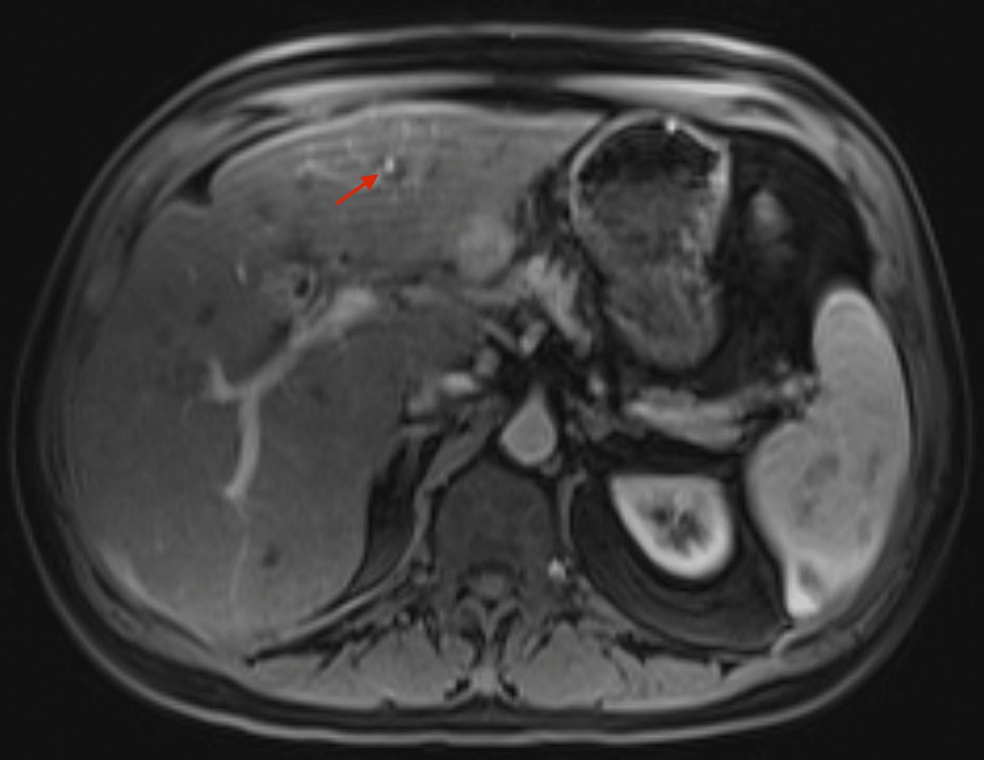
MRI image Magnetic resonance imaging (MRI) revealed an arterially enhancing lesion arising exophytically in segment III of the liver with associated washout and pseudocapsule (red arrow).
